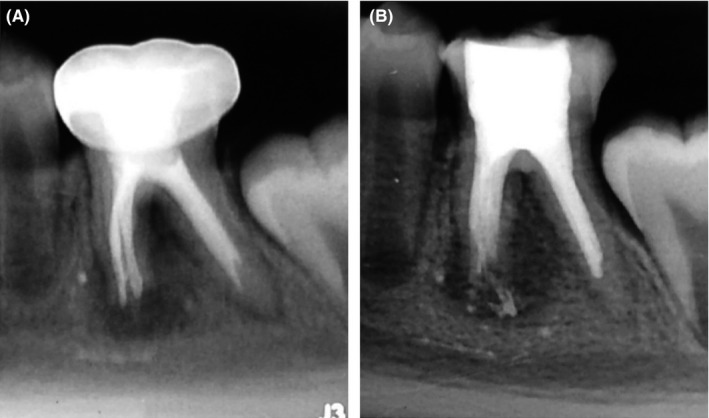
A, Preoperative and (B) postoperative radiographs
